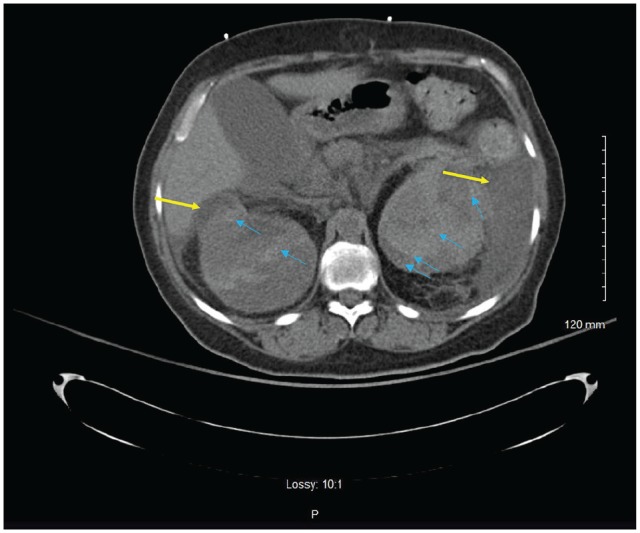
Computed tomography abdomen and pelvis without contrast showing evidence of large bilateral subcapsular renal hematomas (yellow arrows), left greater than right, with attenuation within these hematomas concerning for acute intermittent bleeds (blue arrows).
